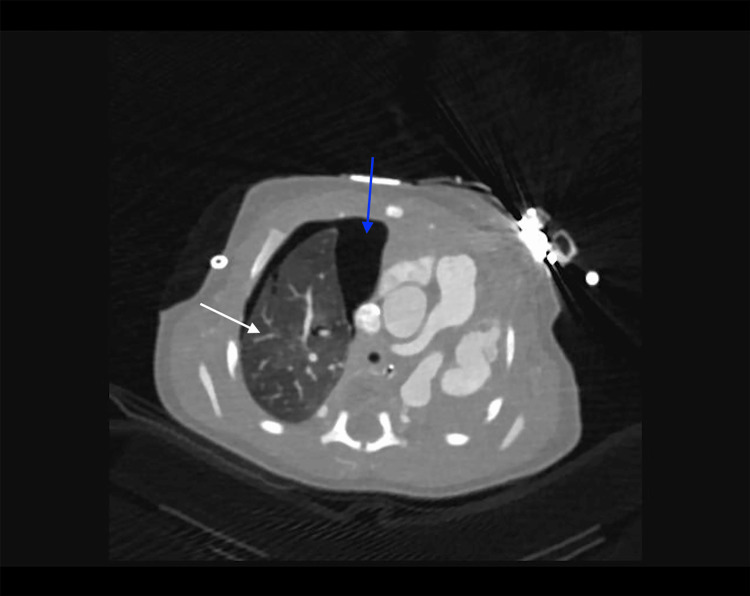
Axial computed tomography image showing complete agenesis of the left lung with associated mediastinal shift to the left The right lung (white arrow) is hyperexpanded and compensatory in appearance. A small right-sided pneumothorax is also present (blue arrow).
